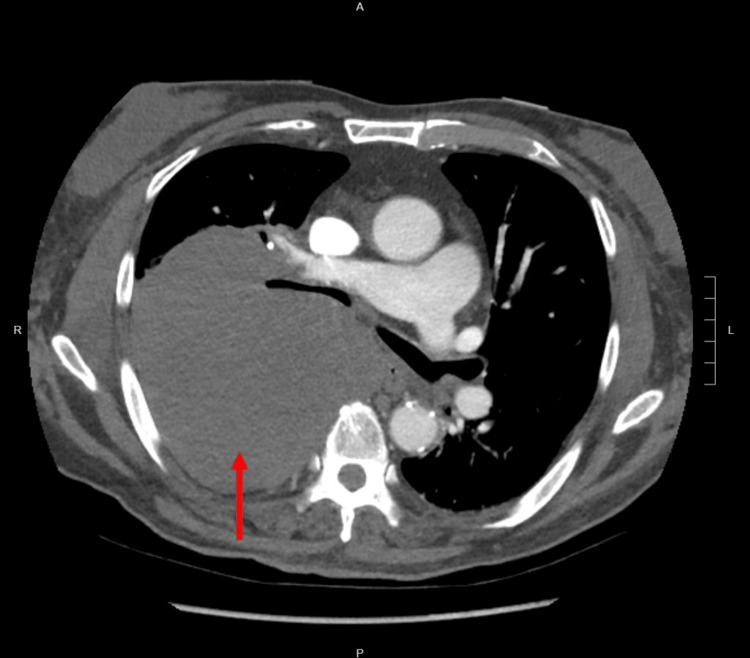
Computed tomography pulmonary angiogram identified a 12.2 cm x 12.9 cm x 16.0 cm mass occupying the majority of the right posterior hemithorax
